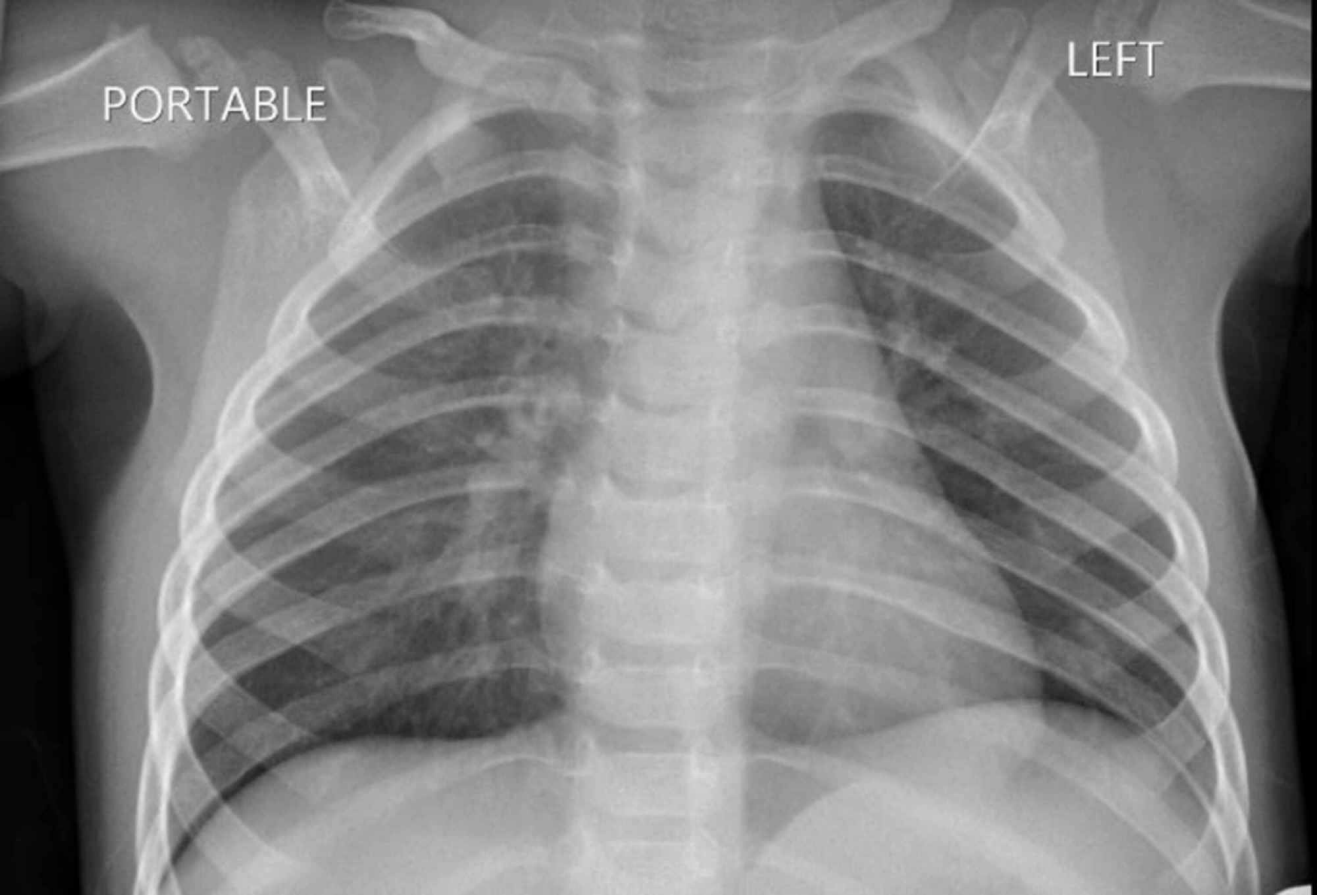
Portable CXR: bilateral interstitial infiltrates more prominent on the right perihilar area, which could represent atypical pneumonia CXR - chest X-ray
